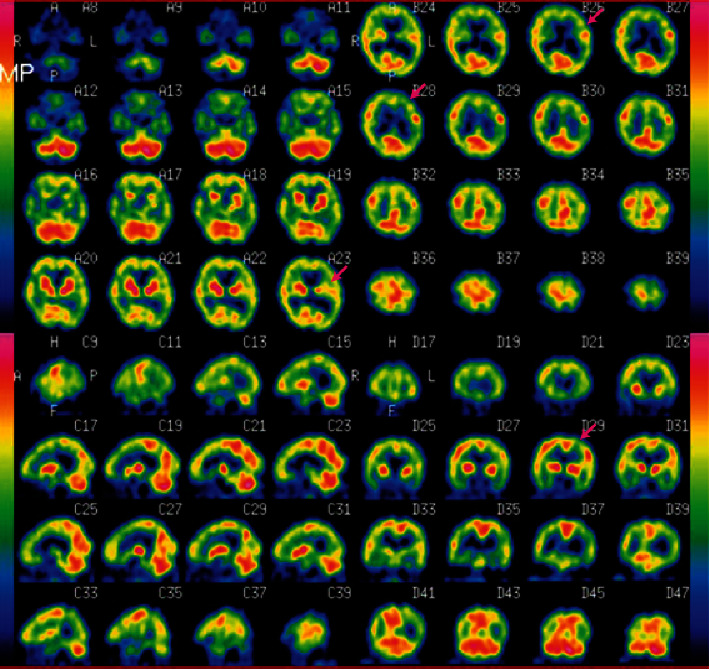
A head image of this patient obtained through single-photon emission computed tomography (SPECT). SPECT detected multifocal hypoperfused areas in the left parietotemporal region and left basal ganglia (red arrows).
